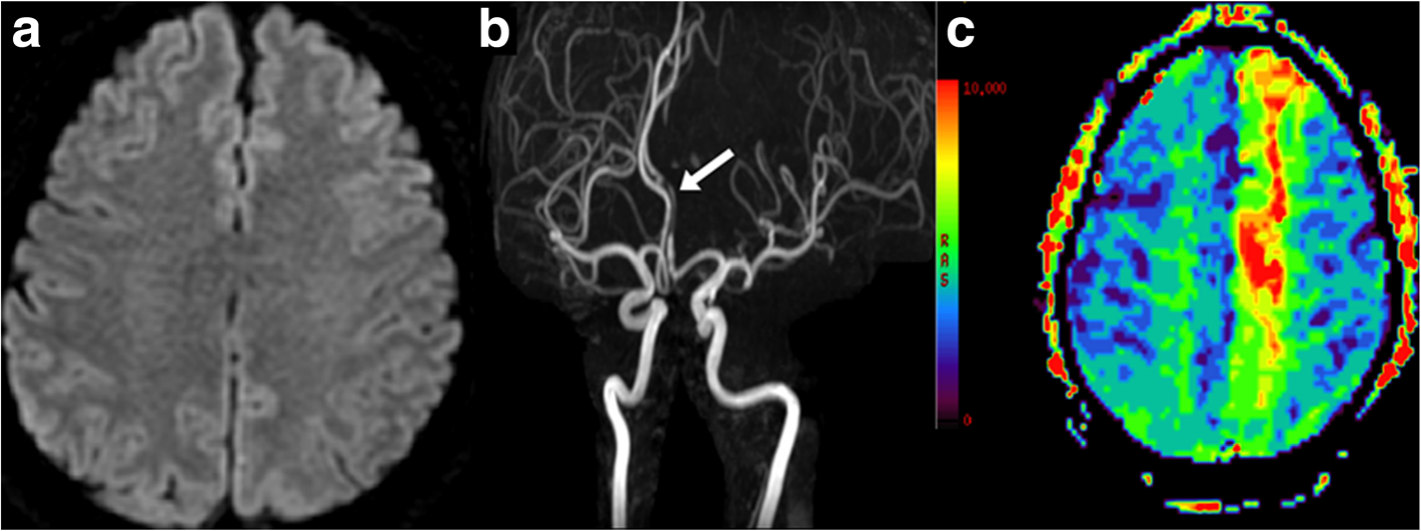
a Diffusion axial brain MRI scan shows no abnormalities. b Cerebral MR angiography shows atherosclerotic narrowing in the left anterior cerebral artery (*arrow*). c Perfusion axial brain MRI scan shows delayed mean transit time in the left mesial frontal lobe
